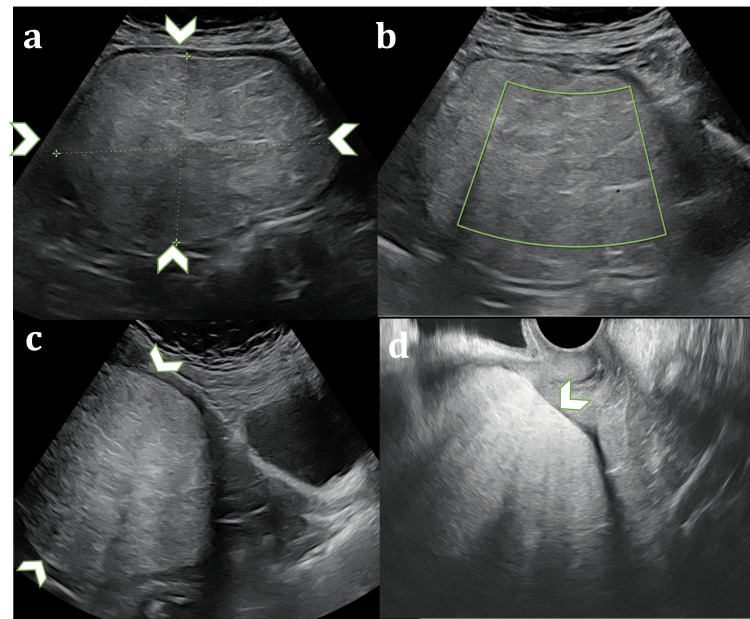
Ultrasonographic features of uterine lipoleiomyoma Transabdominal (a-c) and transvaginal (d) ultrasonography demonstrating a large, well-defined, near-homogeneous hyperechoic lesion (white arrowheads) arising from the uterine fundus, with no significant internal vascularity on color/power Doppler interrogation (b).
